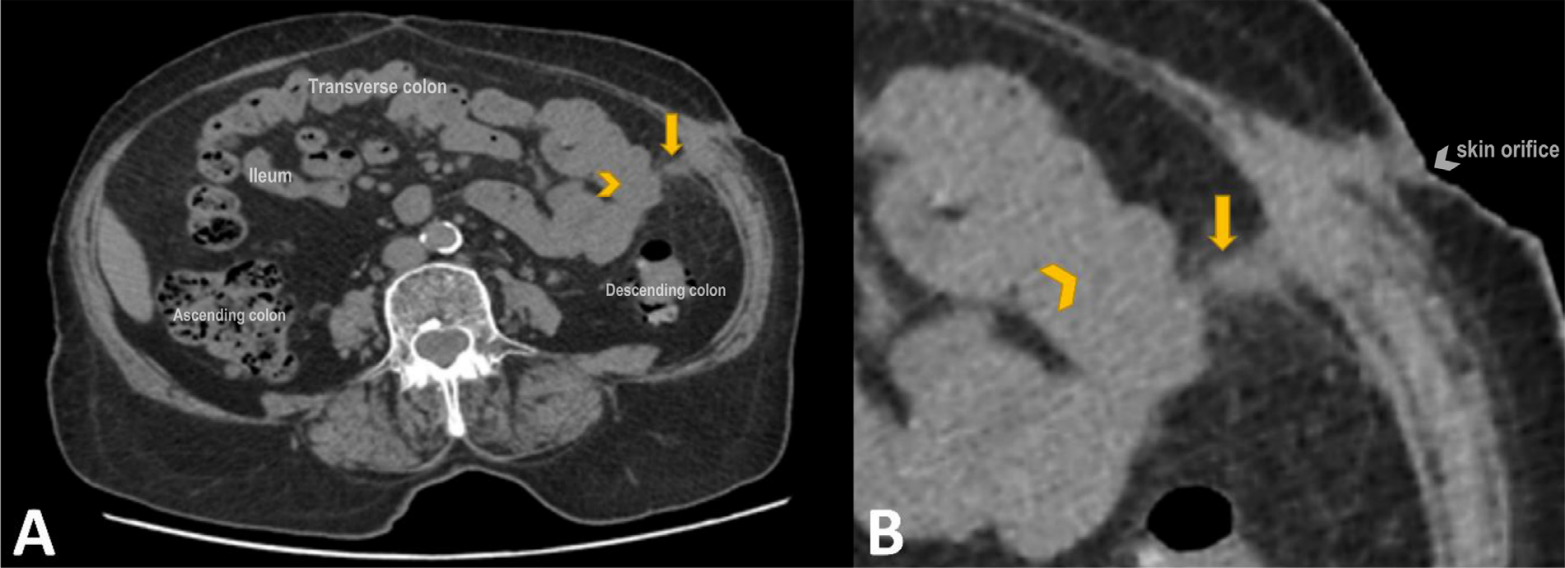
(A) Axial abdominopelvic CT scan (without contrast) obtained after the intervention, showing a fibrotic fistulous tract (arrow) with no evidence of residual peritoneal collections (arrowhead). (B) Magnified view of panel A confirming the successful closure of the fistula. the fistula tract appeared retracted and shorter compared to its preprocedural state, with beading in the tract.
